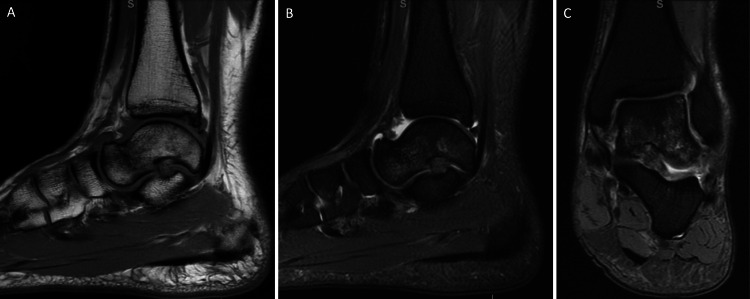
MRI of the left ankle - September 12, 2020. (A) T1-sequence, sagittal view shows a black irregular line at the talar neck demonstrating a possible stress fracture; (B-C) T2-sequence, sagittal and coronal views show signs of bone marrow hyperintensity demonstrating bone marrow edema. The posterior tibial plafond demonstrates a small osteochondral defect, with mild bone marrow edema.
